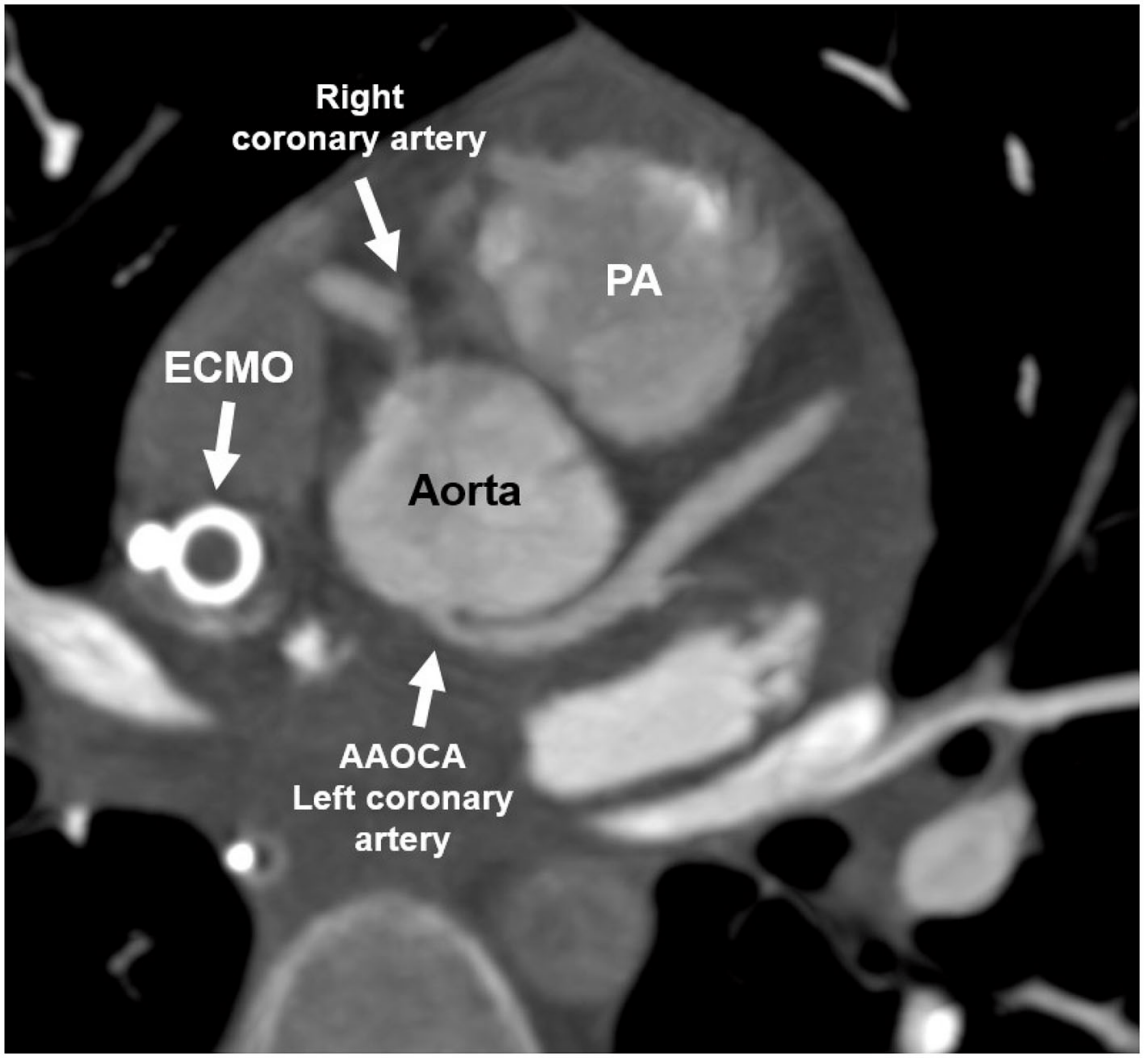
Coronary computed tomography angiography of patient 3: AAOCA with an anomalous origin of the left coronary artery arising from the non-facing sinus without an interarterial course, but with a short intramural course. ECMO, Extracorporeal membrane oxygenation; PA, pulmonary artery.
